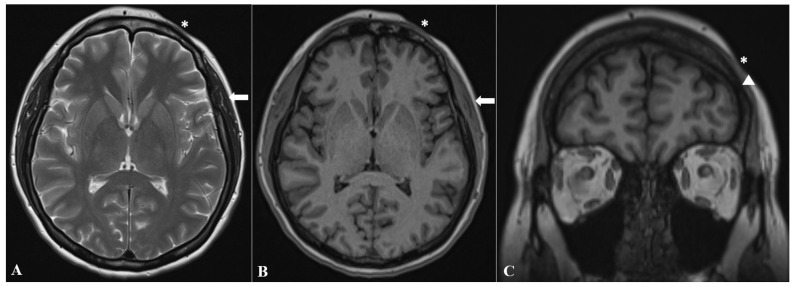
Muscular atrophy (white arrows) and osseous atrophy (white triangle) demonstrated on axial T2-weighted (A), axial T1-weighted (B), and coronal T1-weighted (C) brain MRI sequences. The imaging appearance of the “en coup de sabre” lesion is also visible on these sections, characterized by focal soft-tissue volume loss (white asterisk).
